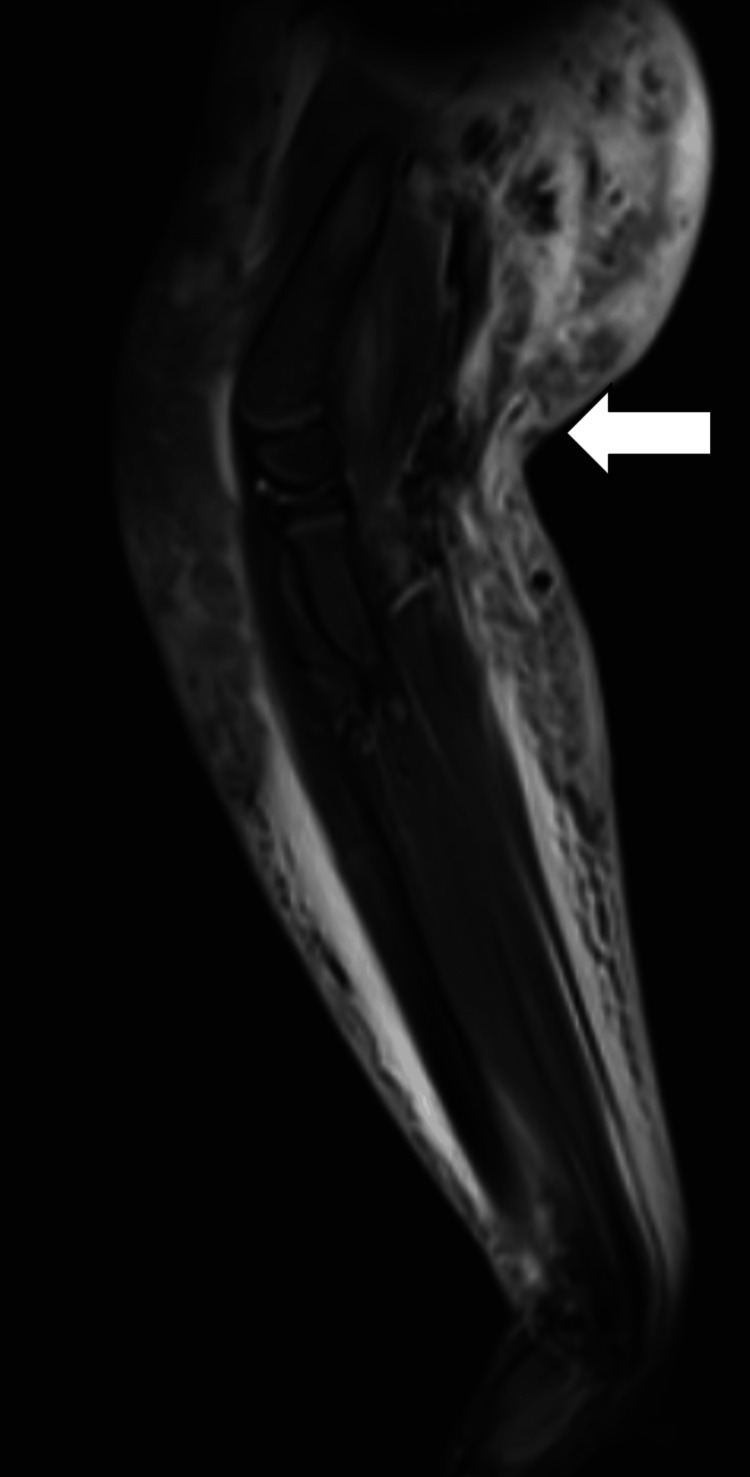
T2-weighted image of the right arm and forearm showing diffuse muscle thickening and subcutaneous interstitial edema (arrow) in all compartments of the right upper limb
